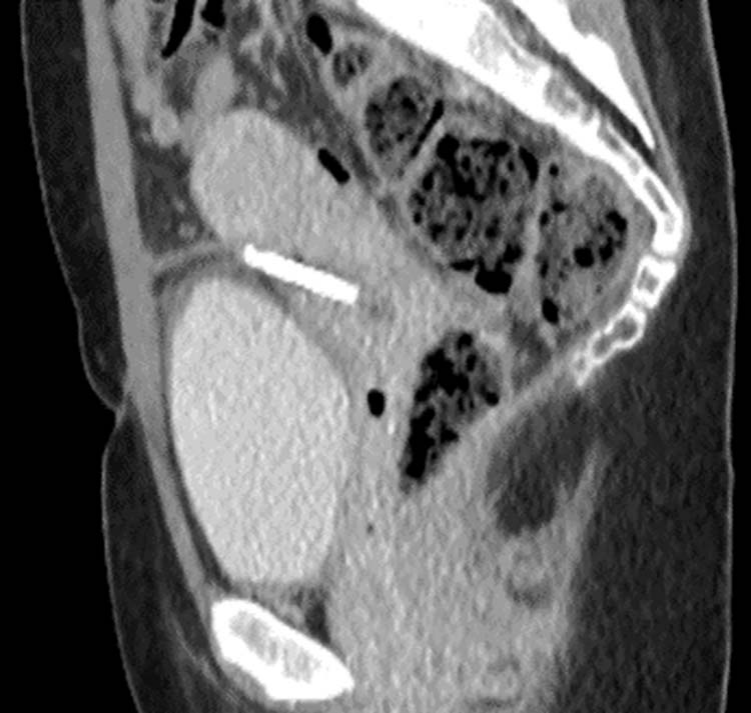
Sagittal CT urinary tract image demonstrating the device lying between uterus and bladder.
